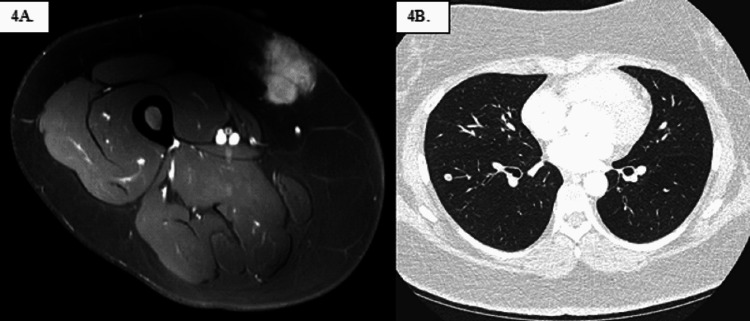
Lung involvement of Rosai-Dorfman disease of the right thigh Magnetic resonance imaging (MRI) with and without contrast (axial view) showed a superficial, solid, contrast-enhancing mass within the right medial thigh, with irregular borders (A). Computed tomography of the chest demonstrated a right lower lobe solitary, non-calcified 8 mm nodule concerning for metastasis of Rosai-Dorfman disease (B).
